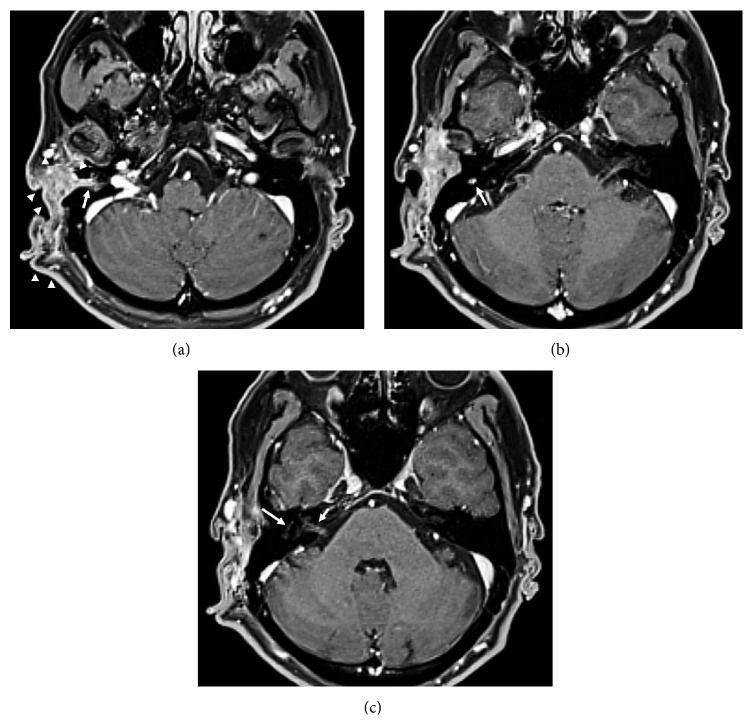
Axial contrast-enhanced T1-weighted MRI after partial resection of the postauricular MPNST. (a) Residual periauricular subcutaneous ill-defined lesions (arrow heads) extending radially from the stylomastoid foramen (arrow). (b) Enhancement of the mastoid segment of the facial nerve (arrow). (c) Enhancement of the tympanic segment (long arrow) and intracanalicular segment (short arrow) of the facial nerve.
